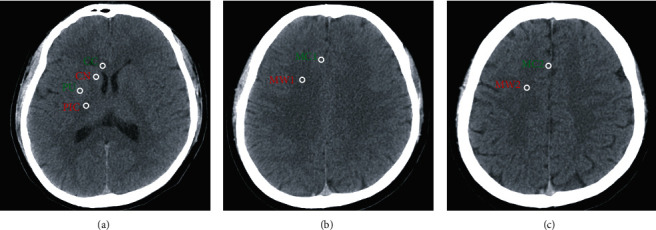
Circular regions of interest were placed bilaterally on brain computed tomography. The regions of interest used for computing the estimation of gray matter to white matter ratio: (a) basal ganglia, (b) centrum semiovale, and (c) high convexity. CC: corpus callosum; CN: caudate nucleus; PU: putamen; PIC: posterior limb of the internal capsule; MC1: medial cortex at centrum semiovale level; MC2: medial cortex at high-convexity area; MW1: medial white matter at centrum semiovale level; MW2: medial white matter at high-convexity area.
